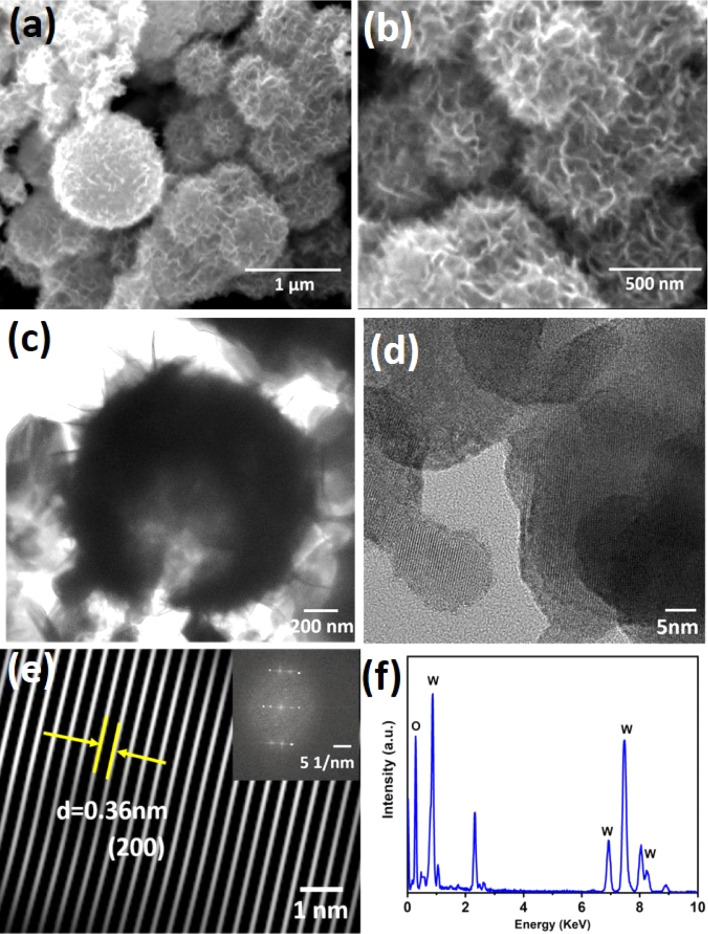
FESEM image of WO_3_ (a) at low magnification and (b) at high magnification. (c) Bright-field (d) high-resolution TEM images of WO_3_. (e) A filtered inverse FFT image showing the lattice plane. The inset shows the FFT (fast Fourier transform) pattern. (f) EDX (energy dispersive X-ray analysis) of the corresponding HRTEM image.
